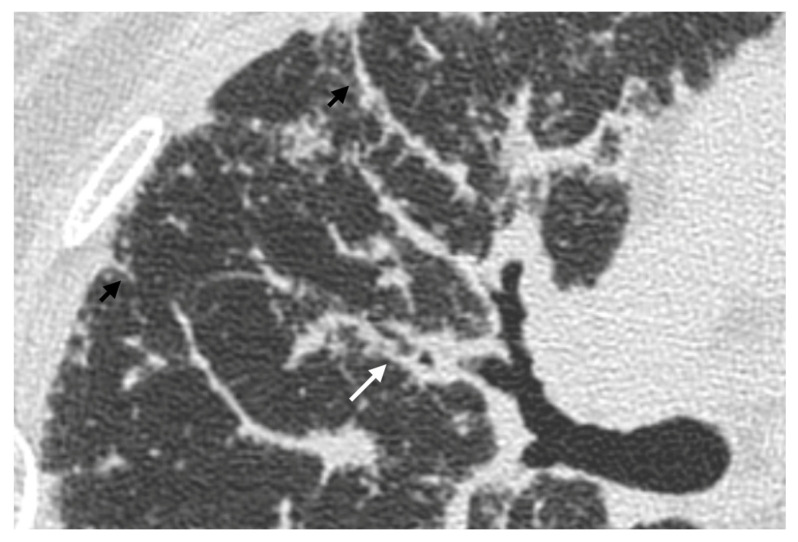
Axial HRCT scan shows diffuse micronodules with a peri lymphatic distribution (black arrows) and a nodular and irregular bronchial wall thickening caused by endobronchial granulomas (withe arrow).
